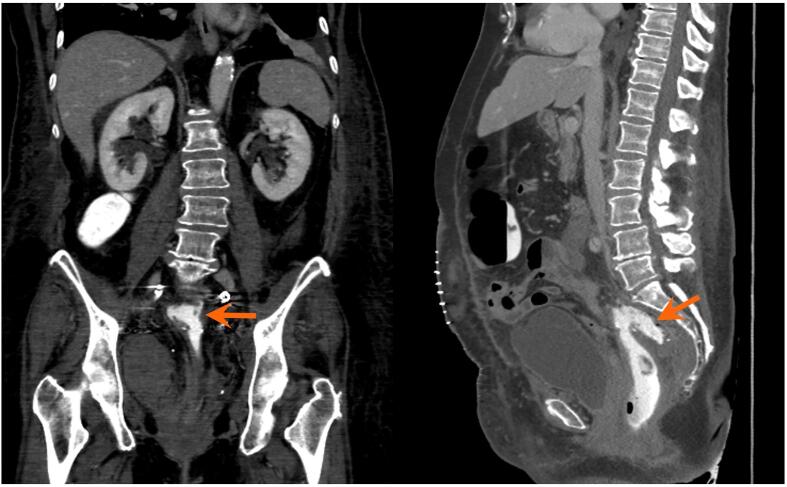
CT with rectal contrast performed a few days later demonstrates extravasation of contrast into this collection (arrows), confirming anastomotic leak.
